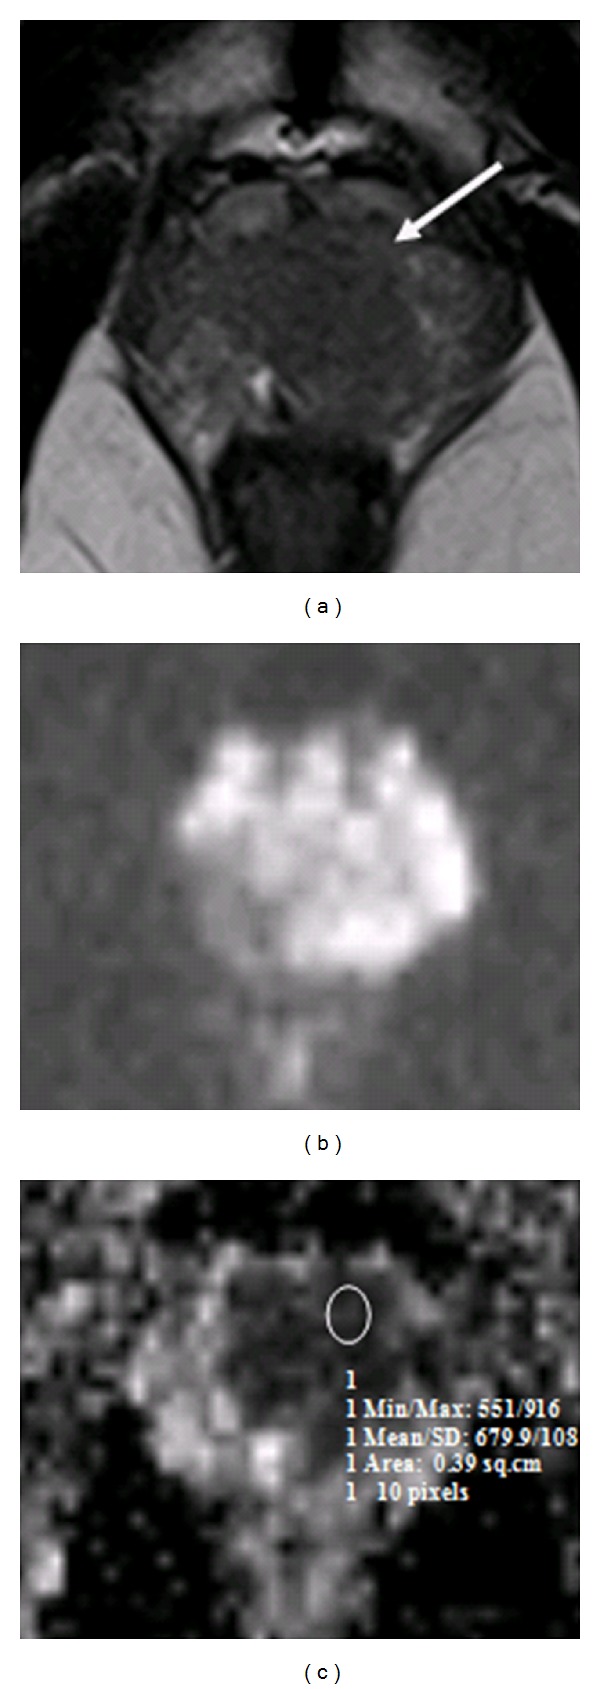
Patient with high-risk prostate cancer. Pelvic T2W transverse image (a) shows a well-defined low signal intensity region in the peripheral zone on the left base (arrow). (b) DWI; (c) an ROI drawn on ADC map shows restricted diffusion in corresponding left base PZ, scored as Gleason 9 on sextant biopsy.
